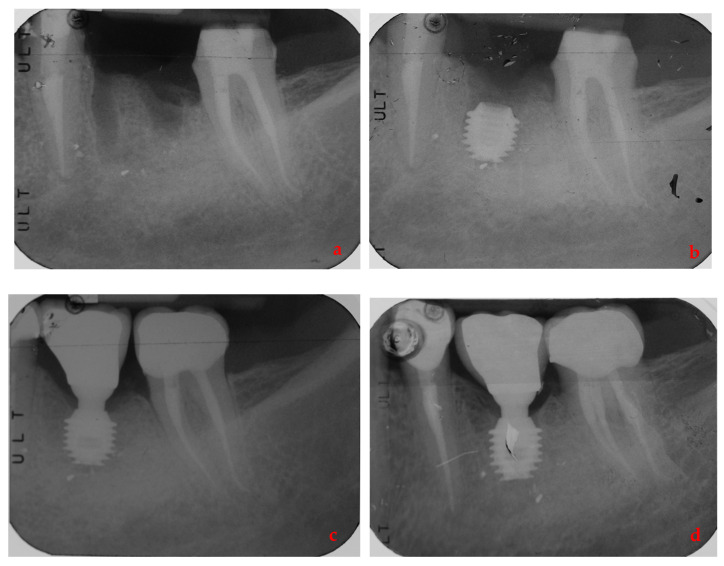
Single implant placed in 3.6 site (5 × 6 mm) of a female patient without history of periodontal disease: (a) pre-operative radiograph before implant placement; (b) radiograph obtained at implant placement; (c) radiograph obtained at time of loading; (d) Radiograph obtained at 3-year follow-up.
